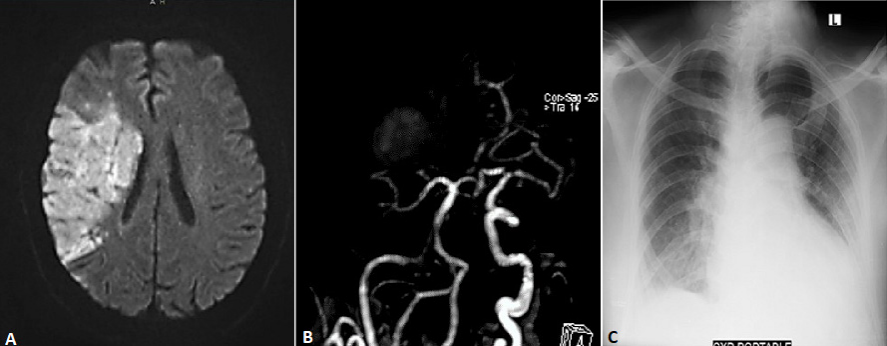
(A) MRI DWI revealed hyperintense signal in the right MCA territory which involved right fronto parietal temporal lobes as well as capsuloganglionic region, indicating abnormal fluid restriction in those areas; (B) 3D TOF MRA revealed extended right internal carotid (intracranial portion) stenosis with near-total occlusion of the right middle cerebral artery; (C) portable chest X-ray shows cardiomegaly and features of interstitial edema seen as bilateral parahilar and lower zone infiltrates
